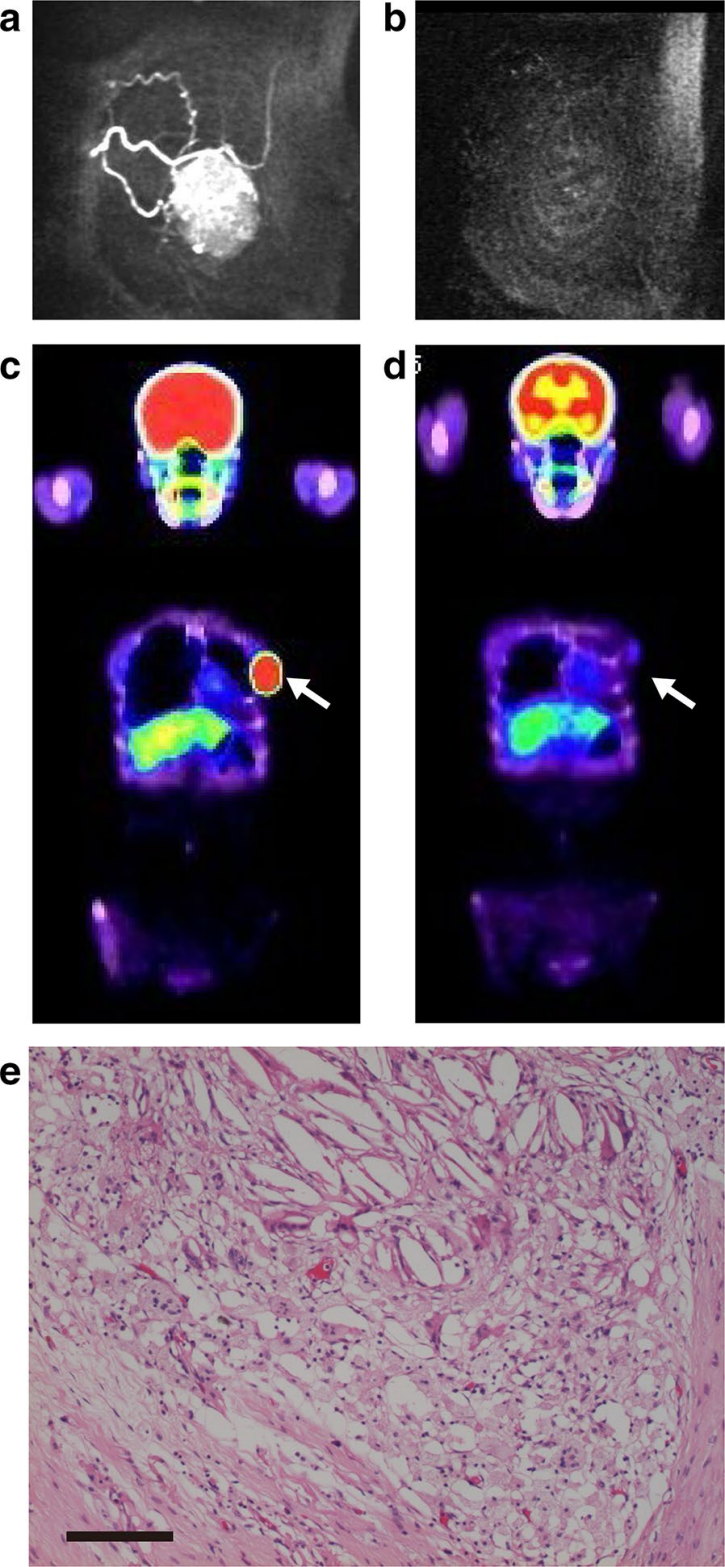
A 55-year-old woman had pCR with abnormal FDG uptake. a The cancer was visualized by a big mass on MRI before NAC. b MRI after NAC; the breast cancer could not be detected, which we considered as a complete clinical response. c FDG-PET/CT before NAC; the SUV_max_ of the primary breast tumor was 14.3. d FDG-PET/CT after NAC; abnormal FDG uptake remained (1.7). e Pathological findings: the cancer cells were gone. *Bar* 100 μm
